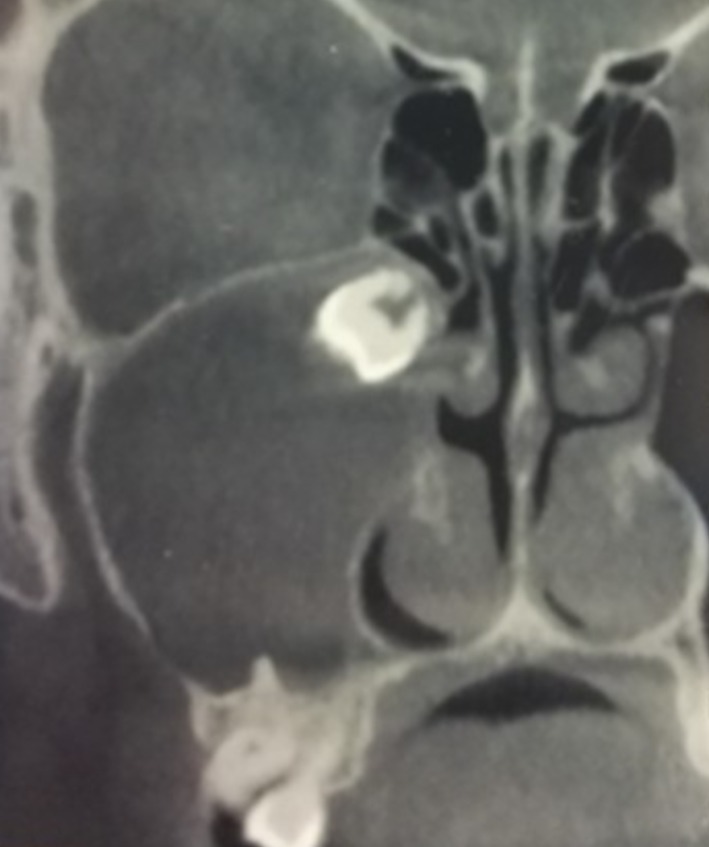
Head computed tomography coronal image showing the dentigerous cysts of the right maxillary sinus, including the tooth germ corresponding to tooth 1.8. A complete opacification of the right maxillary sinus can be observed.
